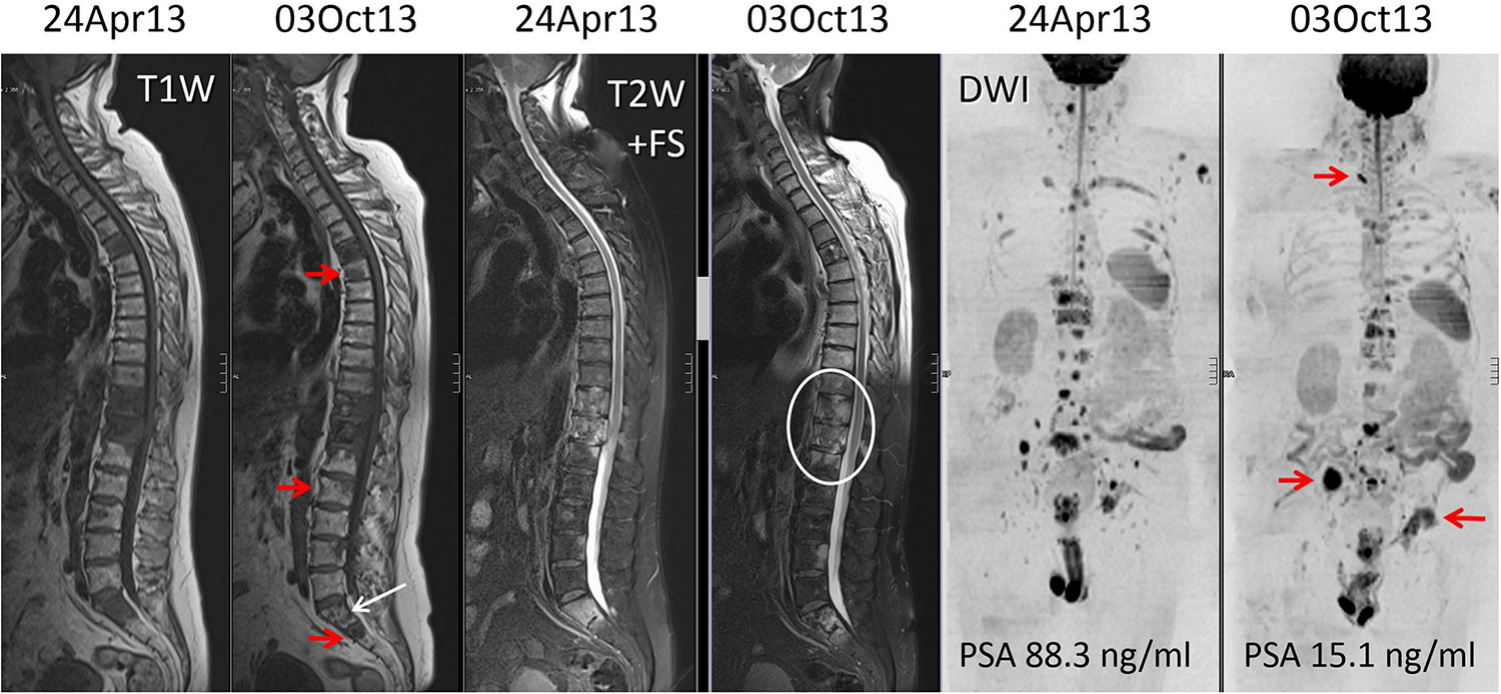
A 64 year old man with metastatic castrate resistant prostate cancer. WB-MRI assessments before and after five cycles of abiraterone therapy. The panel pairs are morphologic T1-weighted (*left*) and fat-suppressed T2-weighted (*middle*) sequences, and high *b* value (*b* 900 s/mm^2^) diffusion-weighted images (*right*) displayed as inverted MIP images. There is a discordant response to therapy documented on the imaging despite reductions in serum PSA levels. The *white arrows* and *ring* show decrease in tumour in the sacrum with return of normal marrow fat and relief of the spinal cord compression on the fat-suppressed T2-weighted sequence. However, the *red arrows* show disease progression in the spine, right iliac bone and *left* acetabulum
